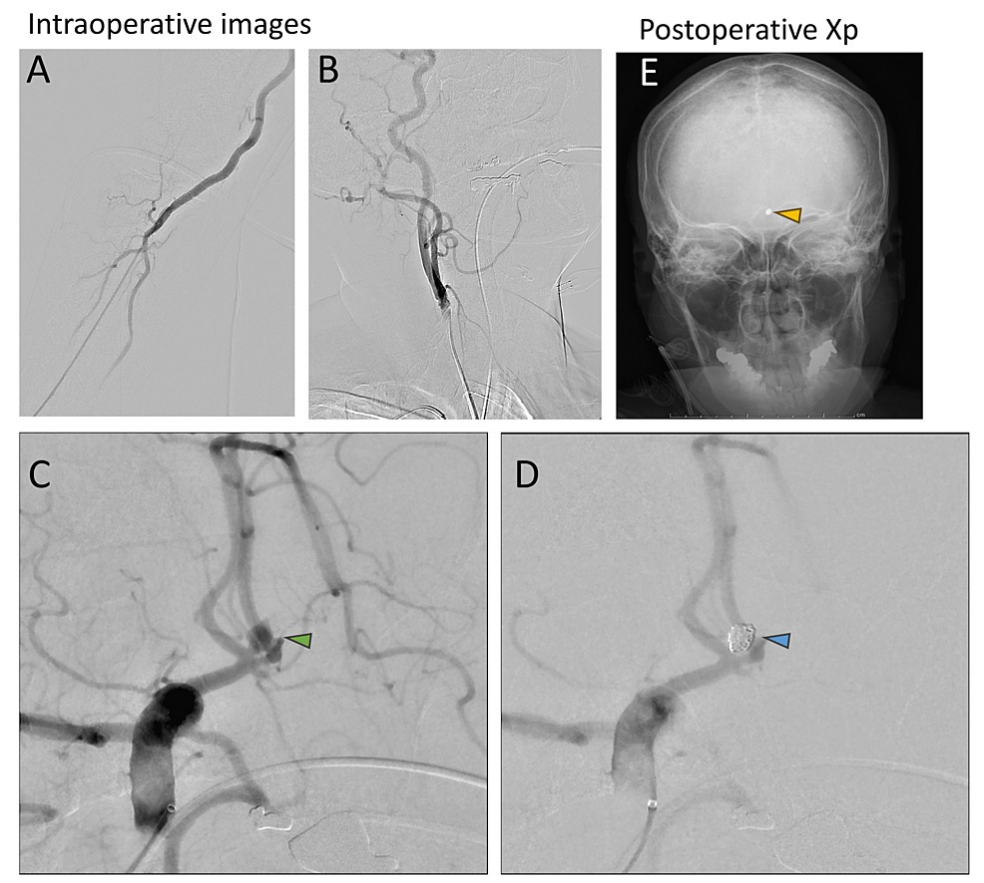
Intraoperative images The patient underwent emergent coil embolization with general anesthesia by radial artery approach (A and B). The aneurysm (green arrowhead in C) was not seen after coil embolization (blue arrowhead in D). Postoperative X-ray confirmed the presence of coils (yellow arrowhead in E)
